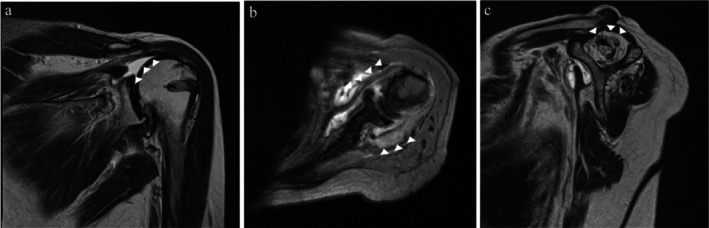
Magnetic resonance imaging findings. (a) Massive tear of the supraspinatus tendon consistent with the Cofield classification (arrowhead). (b) Attenuation and discontinuity of the infraspinatus and subscapularis tendons (arrowhead). (c) Fatty infiltration of the supraspinatus muscle consistent with Goutallier stage IV disease (arrowhead).
